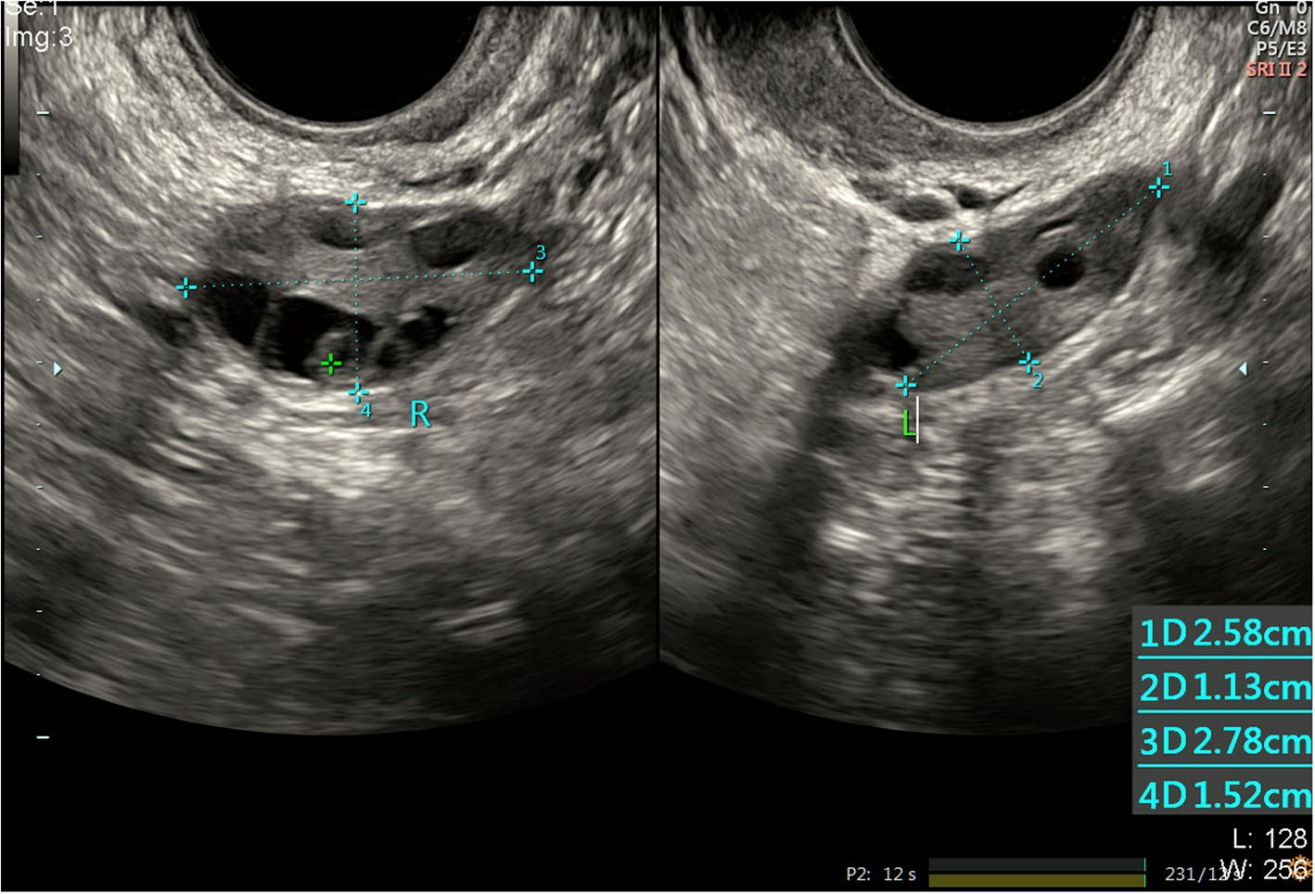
Transvaginal ultrasound scans of the bilateral ovaries. The size of the left and right ovaries was 2.7 × 1.6 cm and 3.2 × 1.4 cm, respectively. The number of antral follicles in the left and right ovary was 12 and 16, respectively, in line with the normal AMH level but in contrast with the high serum FSH and LH levels
